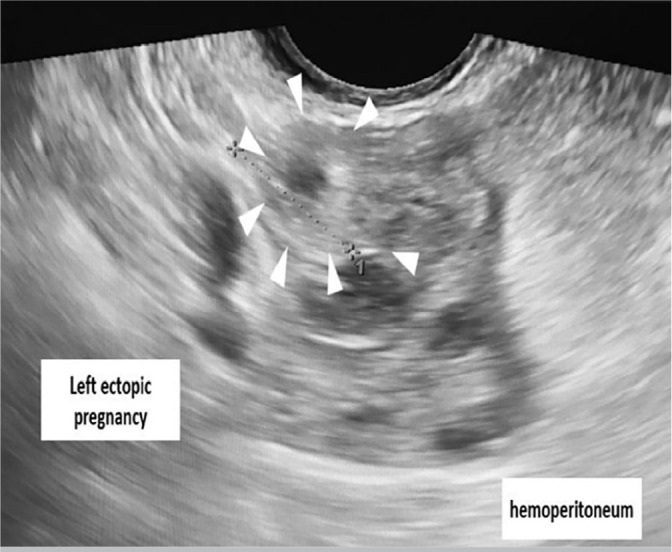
Pelvic ultrasound scan showing right adnexal masses (arrows) measuring
